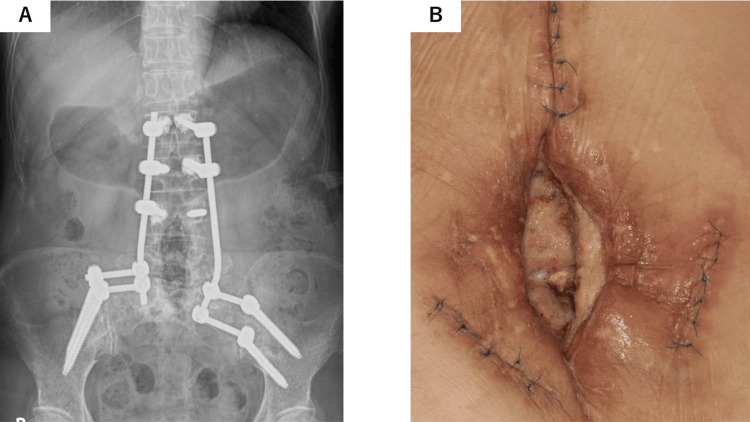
Clinical presentation of the ulceration and exposed spinal fixation hardware. (A) Spinal fixation with pedicle screws in the L1–3 region and iliac screws shown on radiographs. (B) Preoperative view of the refractory ulcer with exposed lumbar fixation hardware in the L4–5 region, showing a 6 cm × 12 cm defect.
